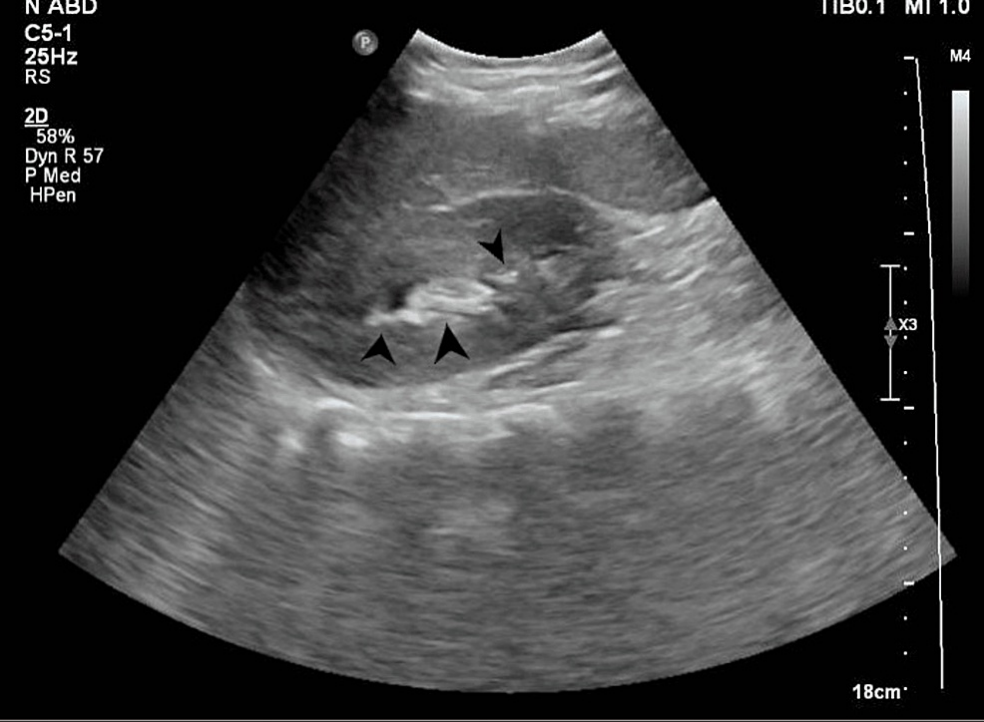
Abdominal ultrasound. Abdominal ultrasound showing a gallbladder with multiple stones (black arrowheads).
